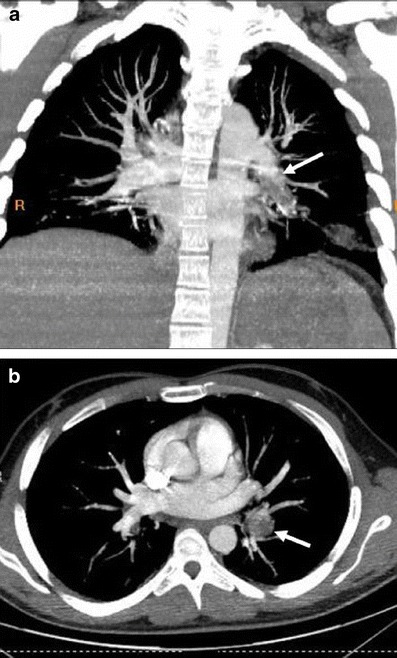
A 31-year-old male with Behçet's disease. Coronal maximum-intensity projection CT image (a) shows thrombosed left lower lobe pulmonary artery aneurysm (*arrow*) and pleural-based, wedge-shaped infarction (*arrowhead*). Axial CT image (b) also shows a thrombosed left lower lobe pulmonary arterial aneurysm (*arrow*)
